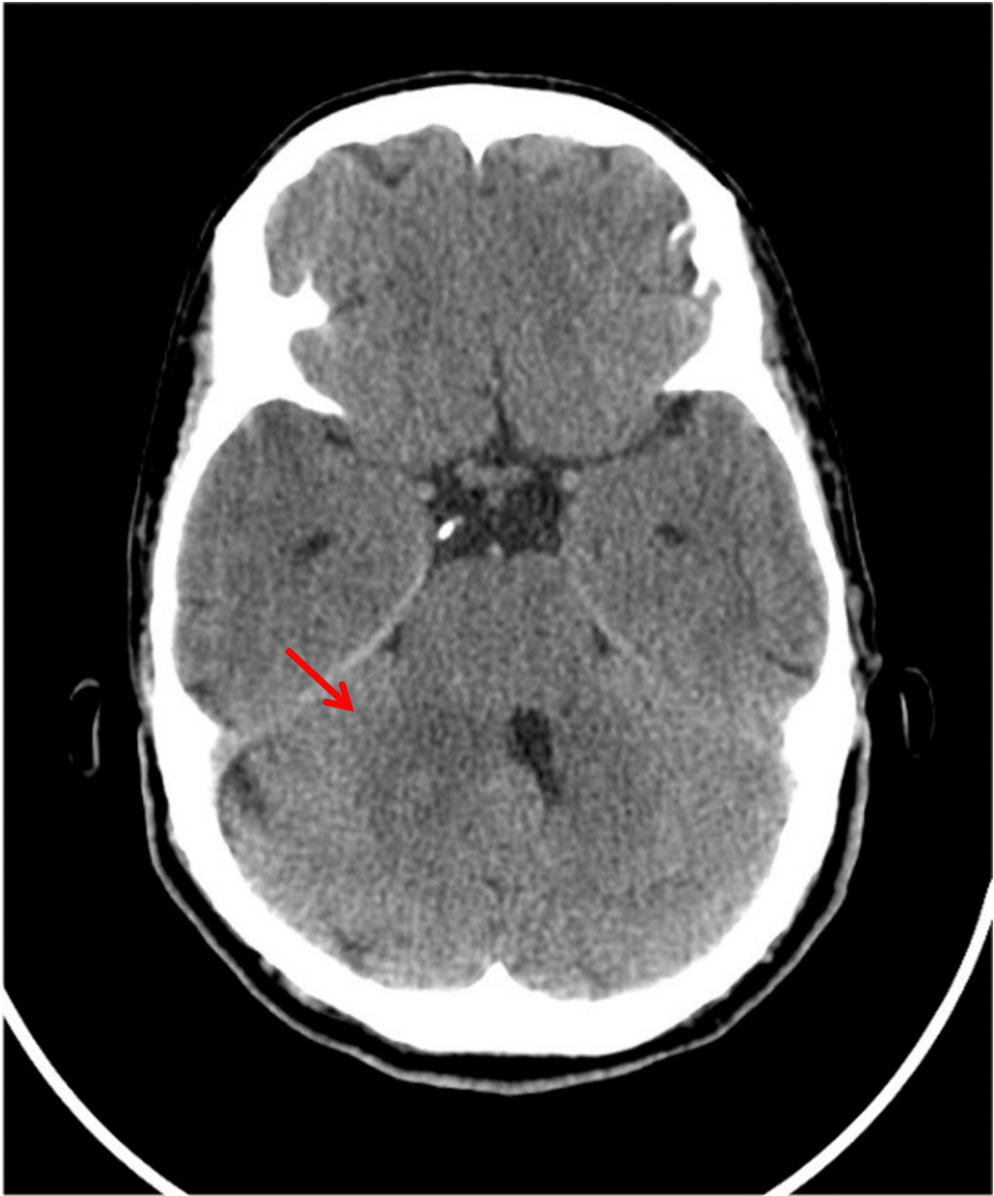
Cranial computed tomography showing a space-occupying lesion involving the right cerebellar hemisphere (red arrow).
